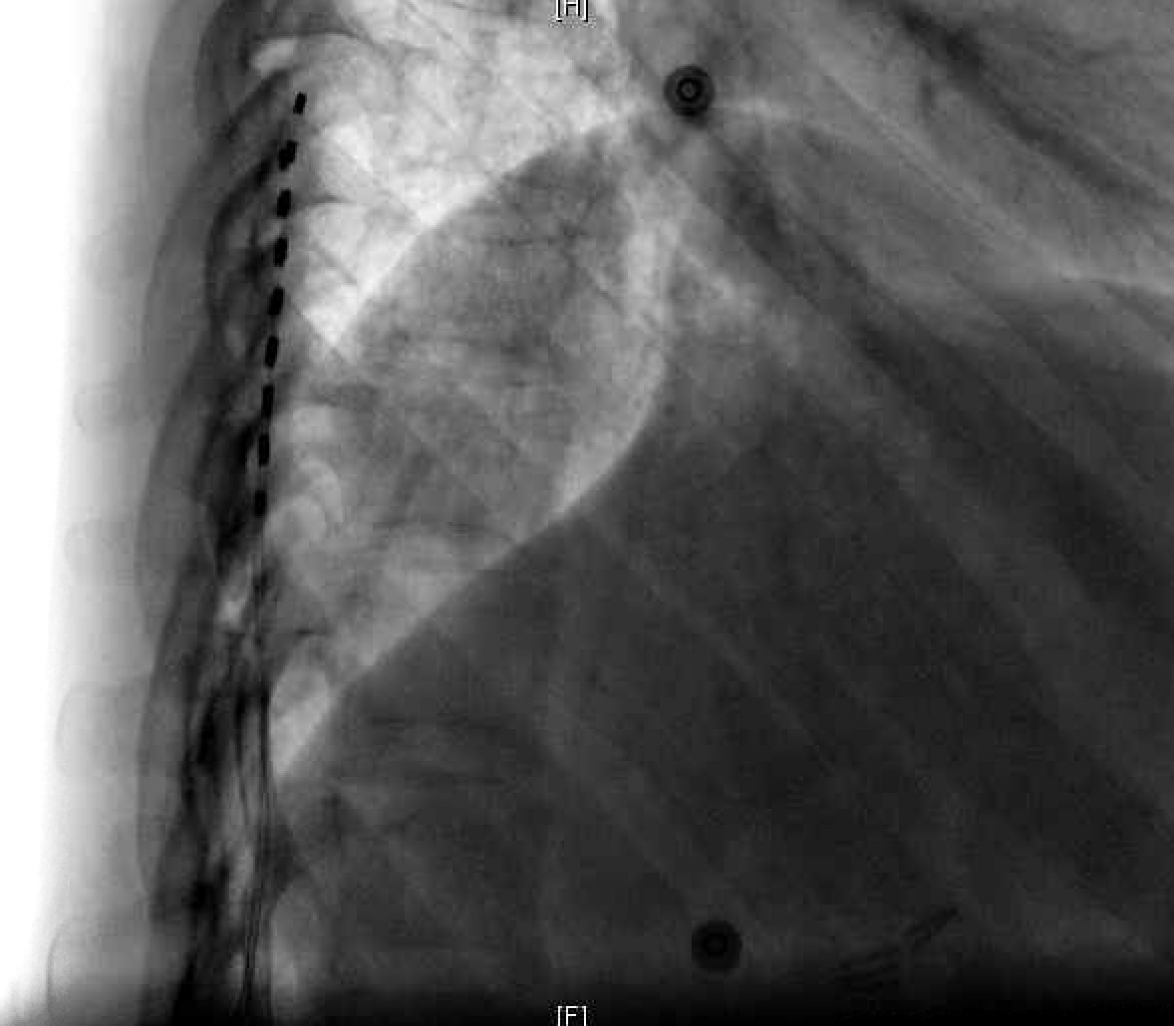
Case 2: Postoperative lateral X-ray showing no evidence of ventral migration.
